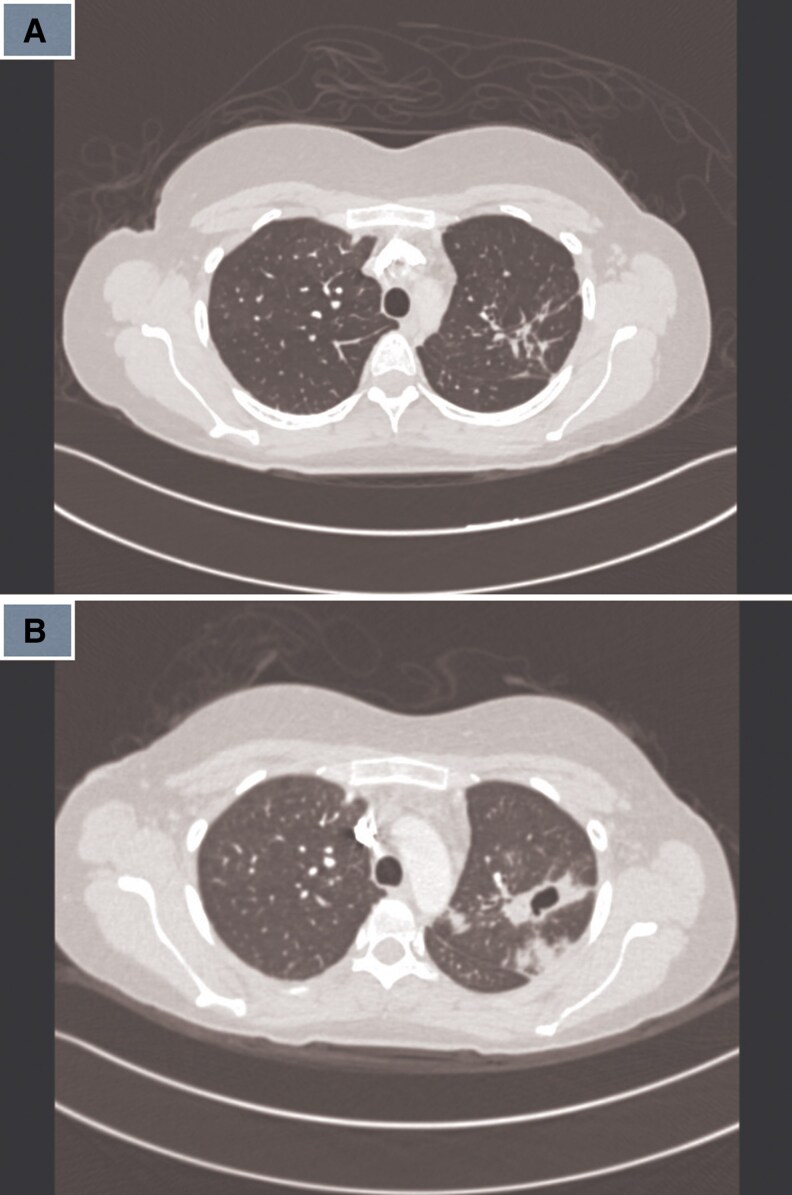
(*A*) Radiological image (eg, CT scan) showing cavitary lesions in a pulmonary *M riyadhense* infection (case 3; Table 2). (*B*) Follow-up imaging showing resolution of cavitary lesions posttreatment (case 3; Table 2).
